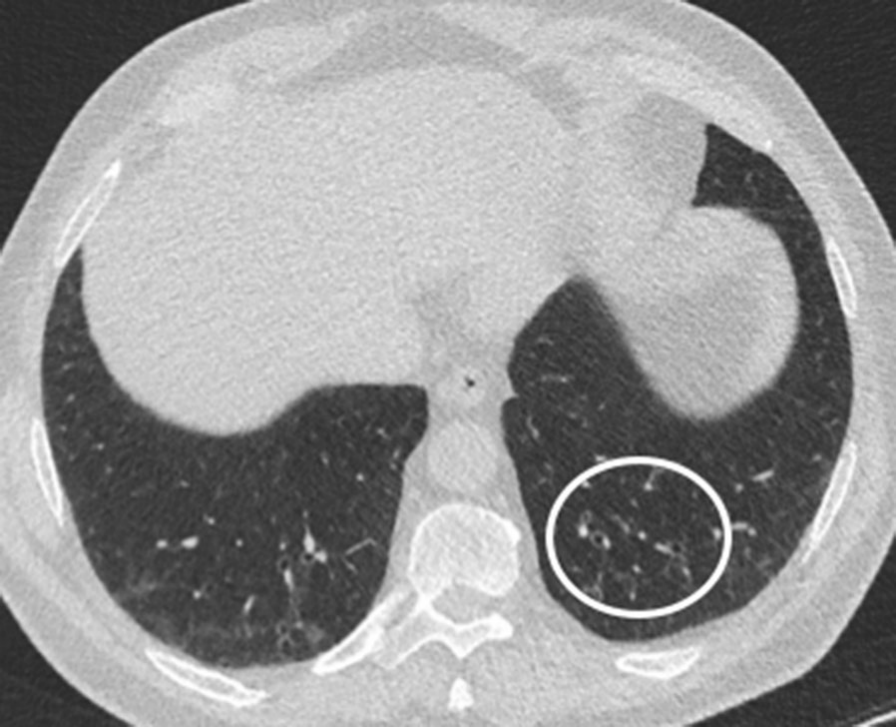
Axial CT image without contrast (slice thickness of 1.5 mm) of a 64-year-old lady shows bilateral GGOs in the lower lobes and fine reticular opacities (*white circle*) with subpleural sparing, compatible with non-subpleural ILAs
